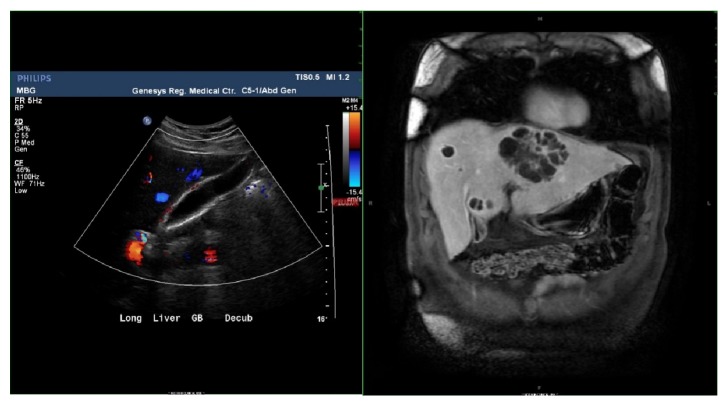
Long imaging showing initial negative ultrasound (left) and follow-up coronal MRCP showing liver abscesses (right).
